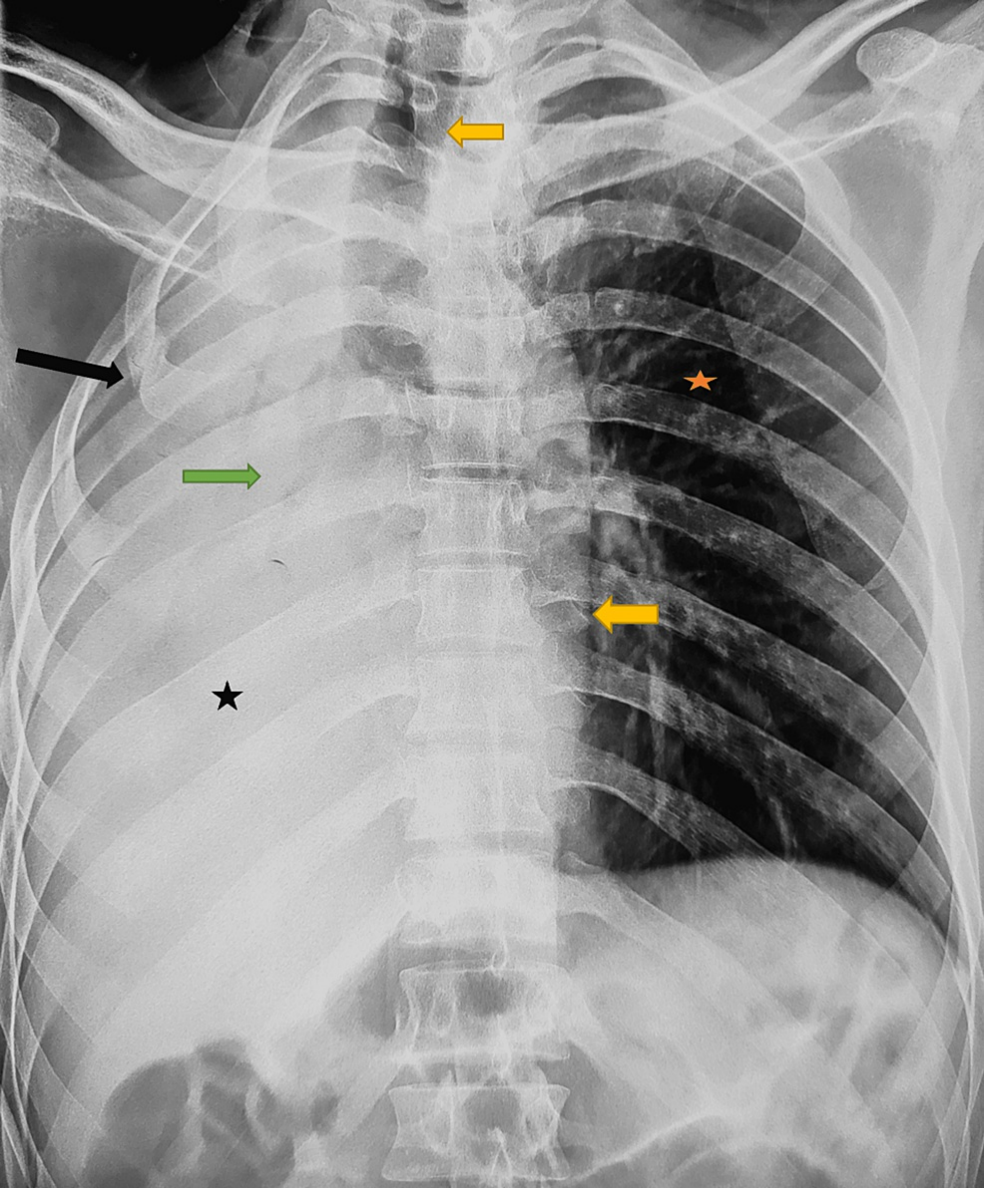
Preoperative chest X-ray, posteroanterior view The image shows diffuse white-out of the right hemithorax (black star), and abrupt cutoff of the right bronchus (green arrow) along with ipsilateral mediastinal and tracheal shift (yellow arrows). The fractured right second rib (black arrow) can also be seen. The left lung appears normal but has compensatory over-expansion (orange star)
